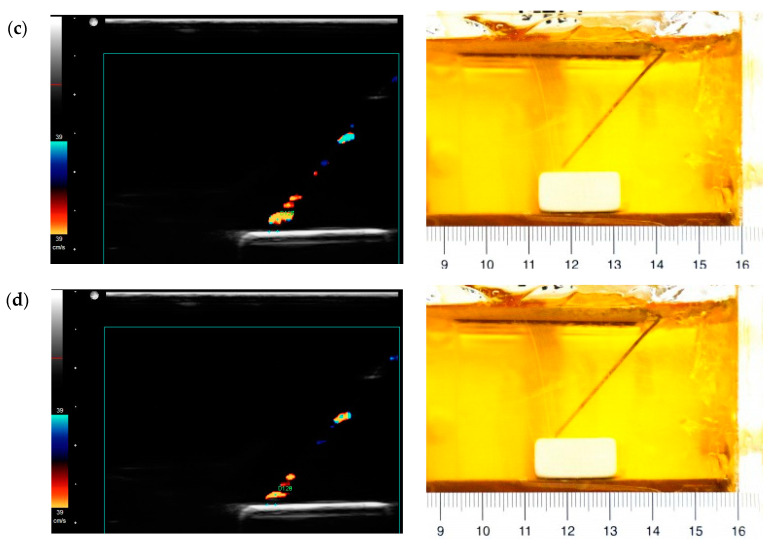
Paired images obtained from the Doppler ultrasound (left) and photographs (right) when the distance is about (a) 1.5, (b) 1, (c) 0.5, (d) 0 mm.
